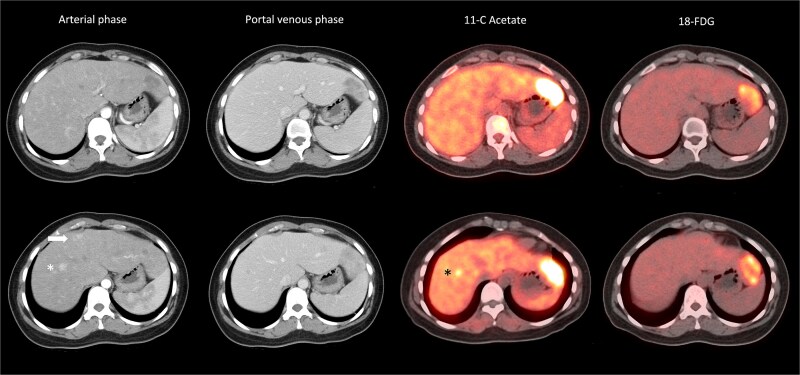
Heterogeneous radiological appearance of the tumours. Lateral S2 tumour was hypodense on arterial phase, and with ^11^C-acetate and ^18^FDG avidity; S4a tumour (arrow) was arterial enhancing but nonavid on PET. S8 lesion (^*^) was arterial enhancing and with ^11^C-acetate but not ^18^FDG avidity.
